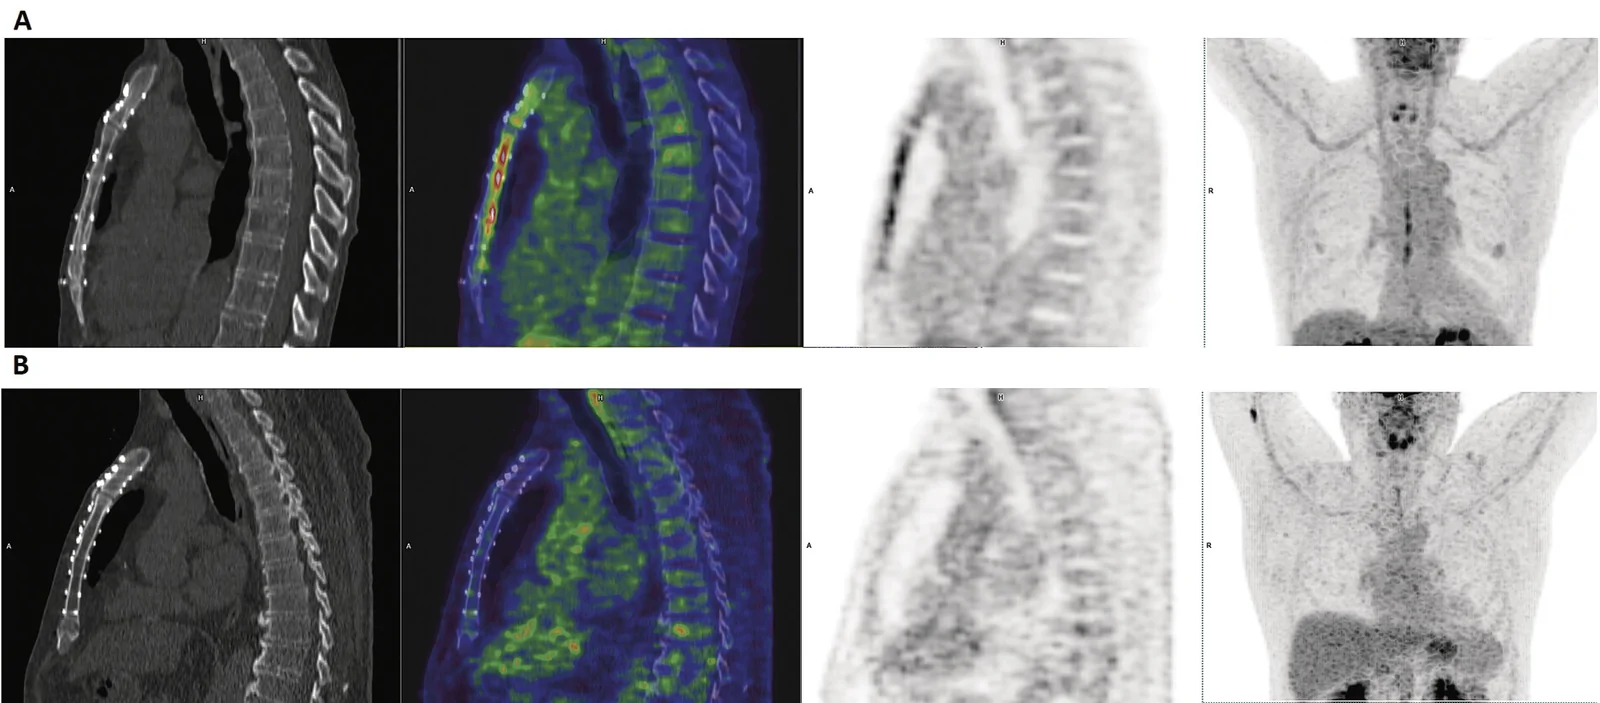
Examples of visually negative and visually positive sternal findings. Sagittal slices (from left to right: CT, PET/CT, PET) and maximum intense projection (MIP) in anterior view (right): Upper row (A): Positive finding in a 71 years old male, 2.1 years after sternotomy (bone wax positive). Lower row (B): Negative finding in a 55 years old male, 2.4 years after sternotomy (bone wax negative).
